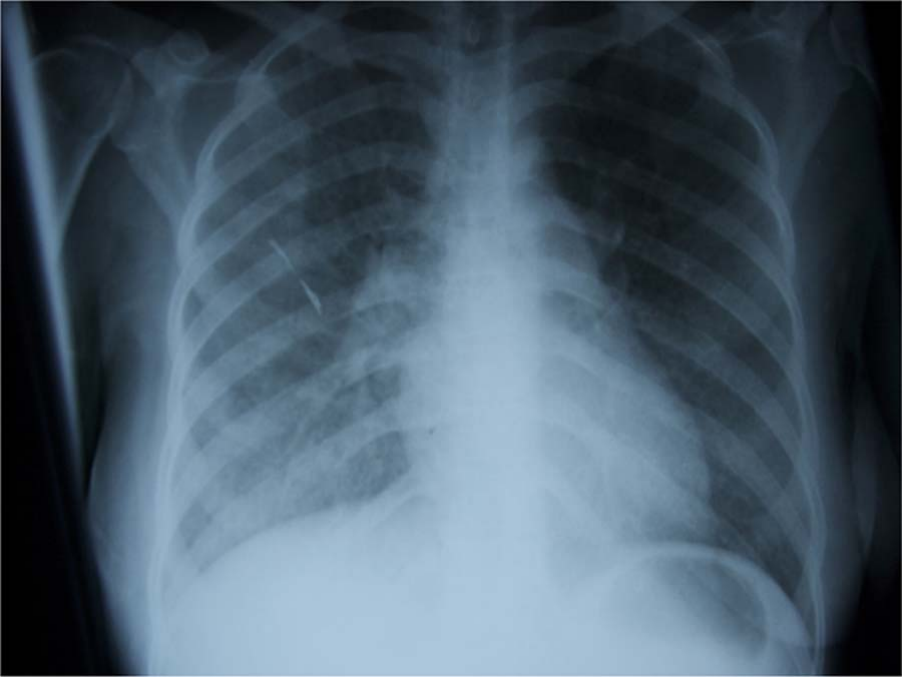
Chest X-ray on Day+1, diffuse interstitial shadowing noted throughout both lung fields, greater on the right.
